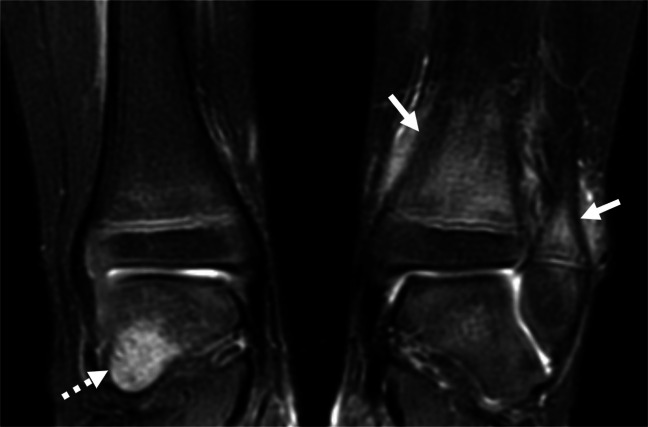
Chronic non-bacterial osteomyelitis (CNO) on MRI. A 4-year-old girl presented with pain and limp. Coronal short tau inversion recovery (STIR) MR image shows areas of edema-like signal within the distal left tibial and fibular metaphyses (*solid arrows*) with adjacent mild periosteal edema. Additionally, there is an area of edema-like signal within the right talus (*dashed arrow*). Other areas seen in the pelvis are not shown
